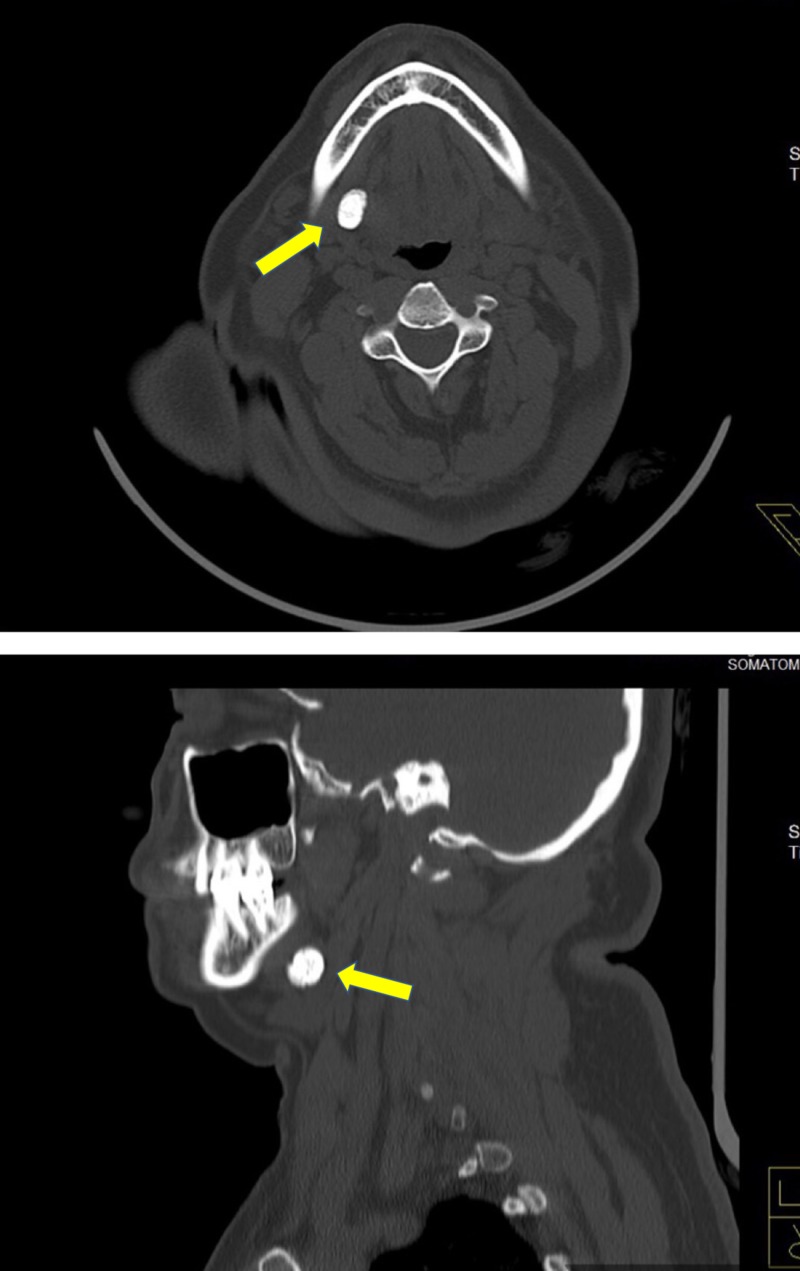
Axial and sagittal view of computed tomography showing sialolith
